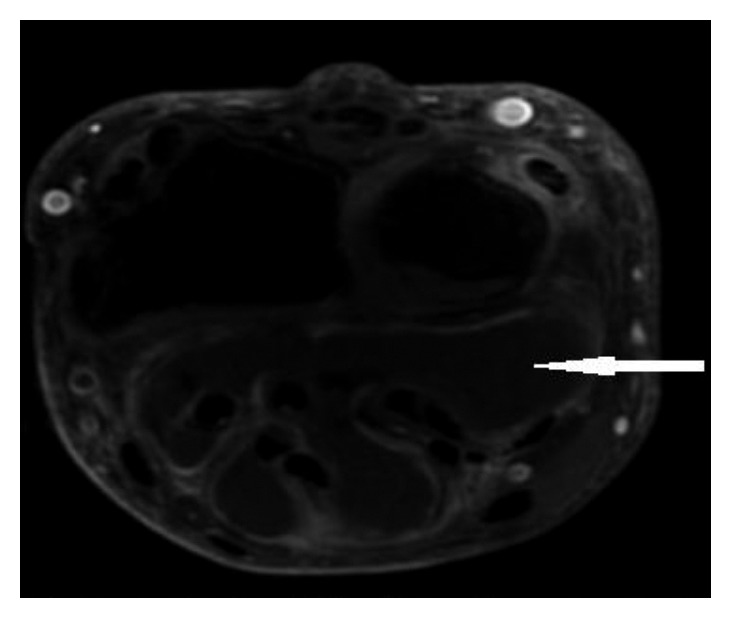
MRI wrist demonstrating tenosynovitis.
